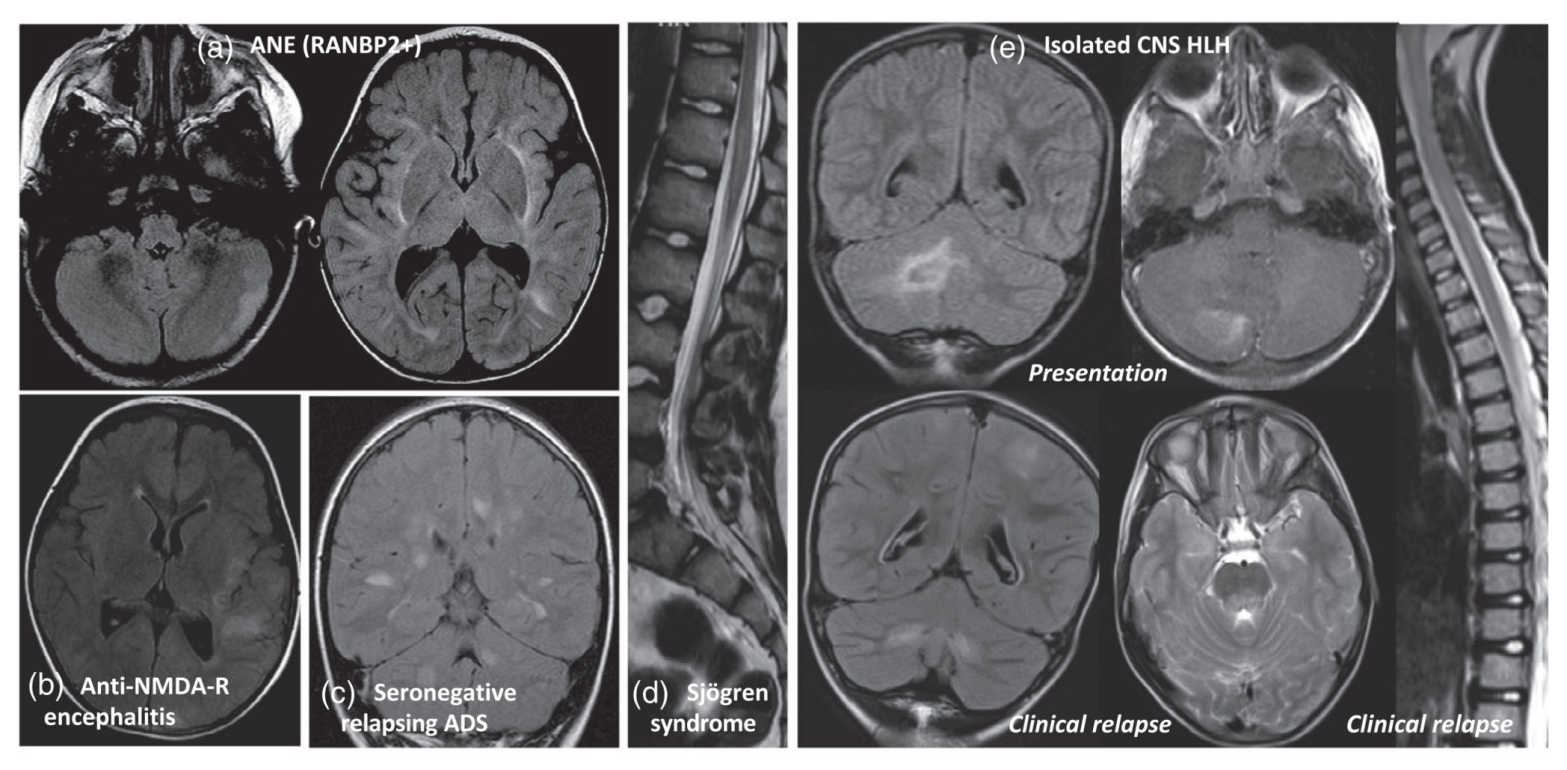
Five cases with alternative diagnoses at 10-year follow-up. (a) A female patient presented at age 16 months with encephalopathy, generalized seizures, abnormal eye movements, and hypotonia. The sister also presented previously with acute disseminated encephalomyelitis at a similar age. Axial T2-weighted fluid attenuated inversion recover (FLAIR) brain magnetic resonance imaging (MRI) showed abnormal hyperintensity of the left cerebellar grey matter and fairly symmetrical hyperintensity of the cerebral white matter, including the external capsules. Genetic screening confirmed *RANBP2* mutation in both siblings. (b) A female patient presented at age 2 years with severe encephalopathy, seizures, and a complex movement disorder. The patient was noted to have a right-sided hemiparesis on clinical examination. The patient was positive for serum anti-*N*-methyl-D-aspartate receptor (NMDA-R) antibodies, and negative for myelin oligodendrocyte glycoprotein-antibodies. Axial T2-weighted FLAIR brain MRI showed an asymmetric distribution of multiple hyperintense grey and white matter lesions, with a notable grey matter predominance. (c) A female patient presented at 14 years of age with encephalopathy, bilateral weakness, sensory loss with cerebrospinal fluid protein elevation (2.6g/L), and positive oligoclonal bands. Axial T2-weighted brain MRI showed an extensive bilateral, asymmetrical, patchy parenchymal signal abnormality involving the deep white matter, brainstem, internal capsules, and cerebellar peduncles. The patient also had longitudinally extensive transverse myelitis (LETM) from C1 to T4 (not shown here). The patient went on to have two further relapses with a similar presentation within the first year. (d) A female patient presented at 12 years of age with bilateral weakness of upper and lower limbs in addition to sphincter dysfunction. Sagittal T2-weighted MRI of the spinal cord showed a lesion in the conus medullaris. (e) A male patient presented at 8 years of age with left convergent squint, ataxia, seizures in addition to lung infiltrates, cycling cytopenia, and hepatosplenomegaly. Coronal T2-weighted FLAIR and contrast enhanced T1-weighted brain MRI at presentation showed a heterogeneously enhancing lesion in the right cerebellar hemisphere bearing some localized oedema and leptomeningeal enhancement. Follow-up imaging on relapse showed symmetrical hyperintense lesions on T2-weighted images in the cerebellum and dorsal pons, symmetrical lesions in the cerebral hemispheres as well as an LETM. The patient underwent a bone marrow transplant with an unsuccessful central nervous system response with clinical and neurological evidence of progression that led to death.
